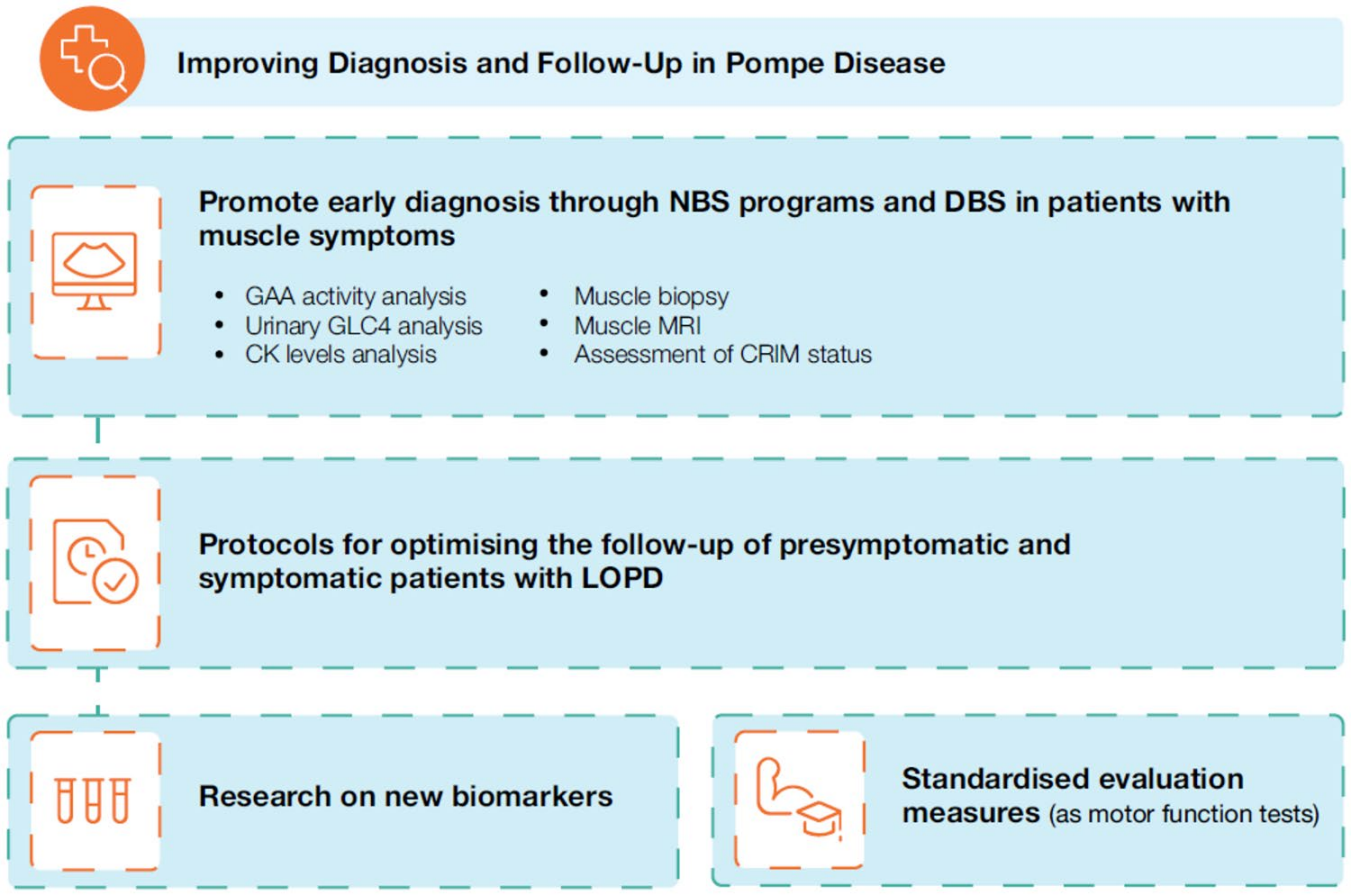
Diagnostic and evaluation recommendations for a centre of excellence for Pompe disease. *CK* Creatine kinase; *CRIM* Cross-reactive immunological material; *DBS* Dried blood spot; *GAA* Acid alpha-1,4-glucosidase; *GLC4* Glucose tetrasaccharide; *LOPD* Late-onset Pompe disease; *MRI* Magnetic resonance imaging; *NBS* Newborn screening
